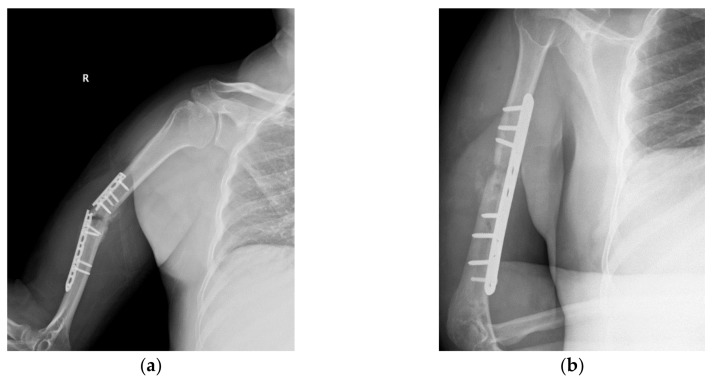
Clinical details: (a) X-ray: implant failure with subsequent plate breakage; (b) X-ray post-operative control after revision.
